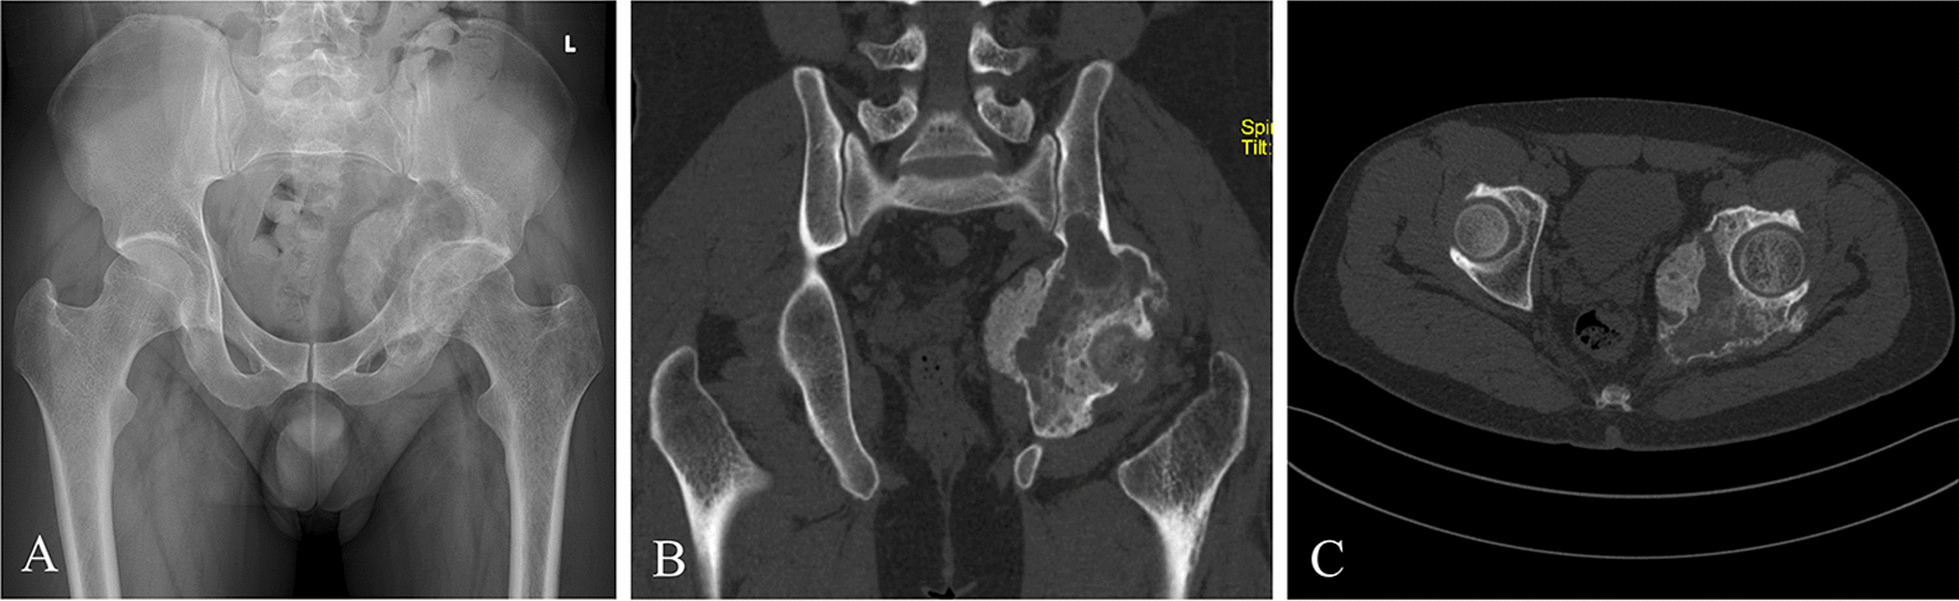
Preoperative images of a 29-year-old patient. Anteroposterior X-ray (A) and CT scans (B, C) showed that the lesion centered on area II Enneking, but with extension including areas I and III
